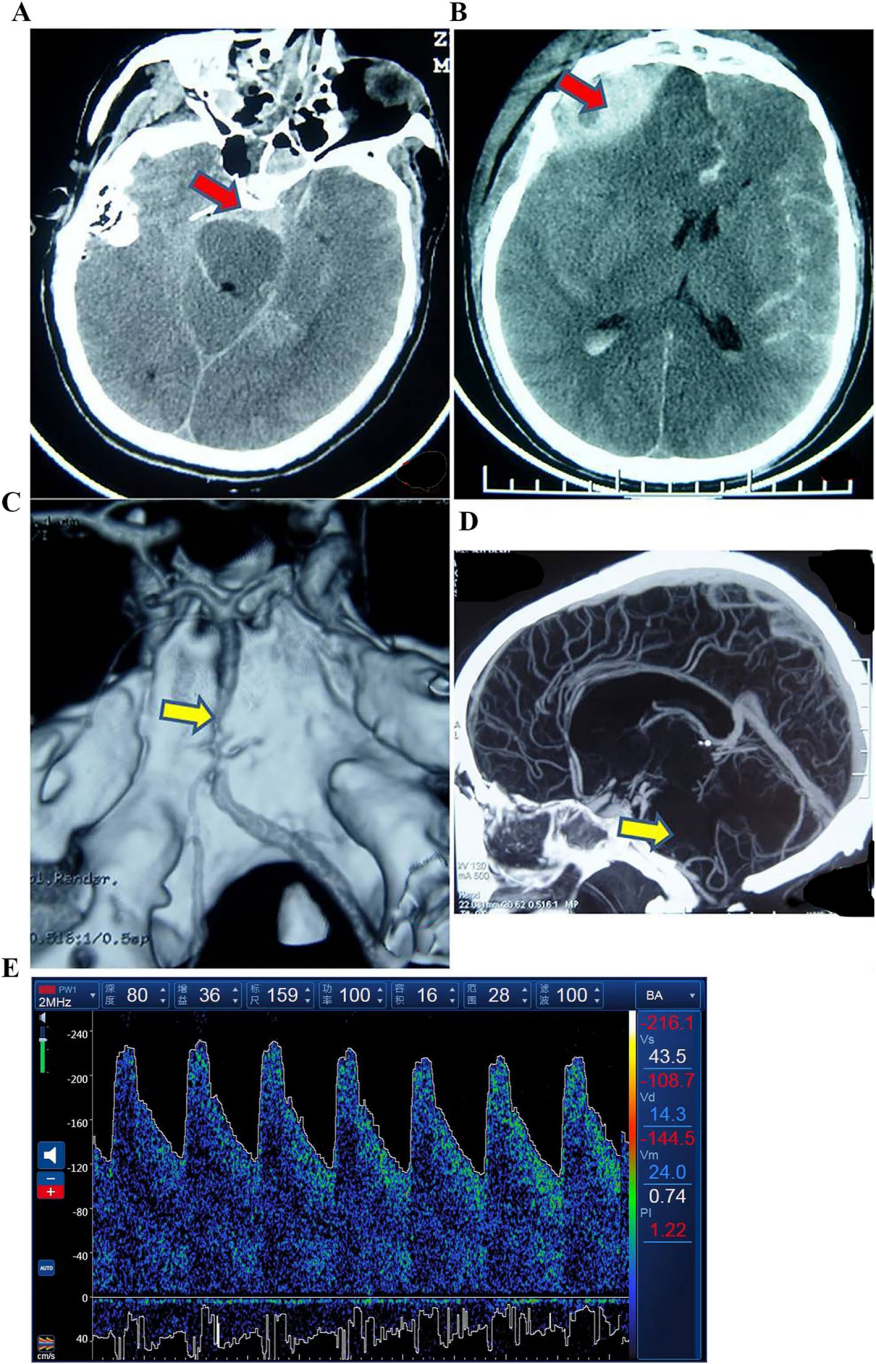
CVS image of CTA. A case of a severe TBI patient with GCS 5. (A) and (B) indicate epidural hematoma and traumatic subarachnoid hemorrhage (red arrow) by CT; (C) and (D) indicates CVS of the basilar artery and vertebral artery (yellow arrow) by CTA; (E) TCD showed CVS of the basilar artery.
